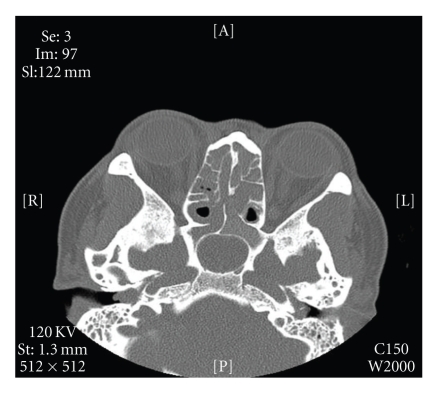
Axial computed tomographic scan demonstrates bilateral aural polyps with thickened sinonasal mucosa.
